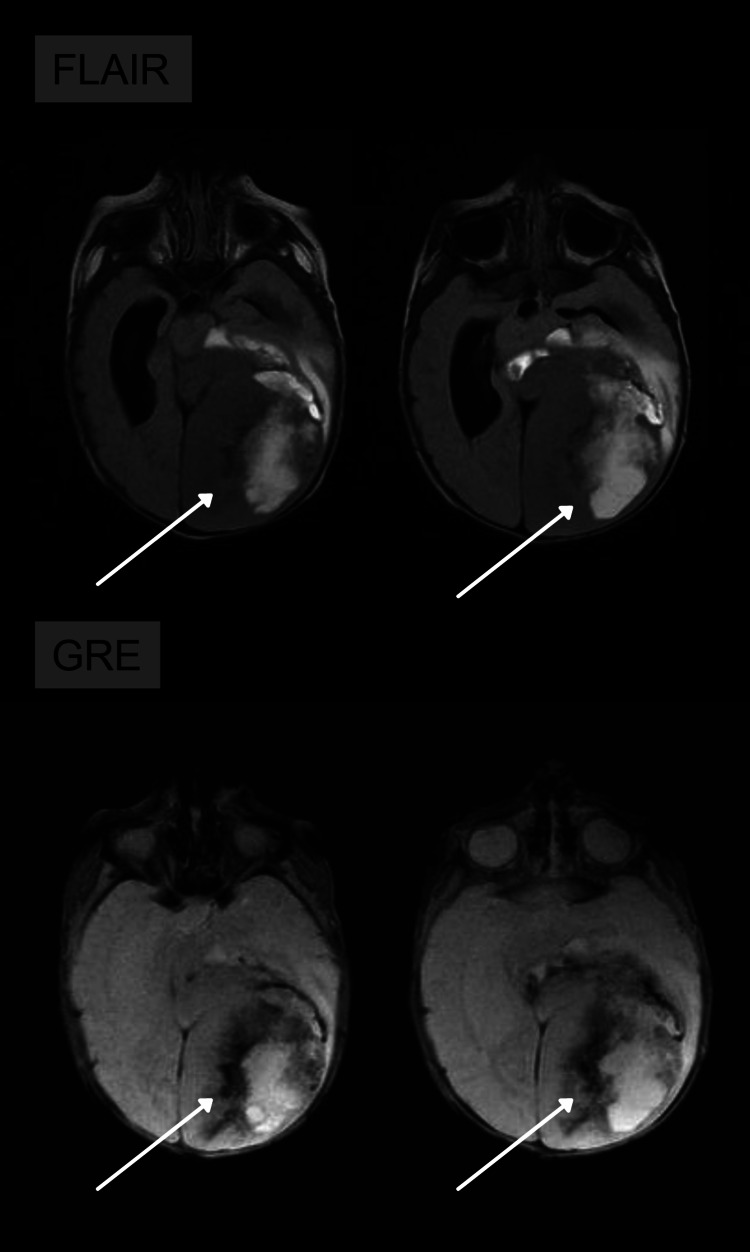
FLAIR: axial images in the FLAIR sequence show an increase in diffuse signal both in the tumor area and in the edema of the white substance, indicating a high degree of hydration of the lesion. GRE: axial images in the GRE sequence showing areas of hypointense signal, which may correspond to calcification or hematic component. GRE: gradient-echo sequences; FLAIR: fluid-attenuated inversion recovery
